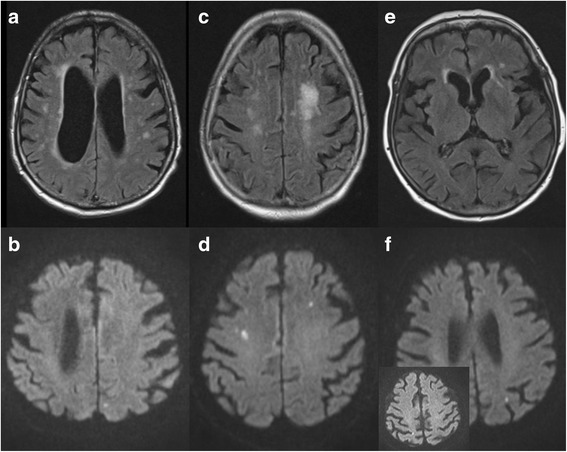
Brain magnetic resonance imaging of three patients during septic shock. The images are fluid-attenuated inversion recovery (FLAIR; a, c, and e) and echo planar imaging diffusion-weighted imaging (DWI; b, d, and f) scans. a and b An 81-year-old male patient with urosepsis. a Axial FLAIR image obtained on day 5 after the onset of septic shock shows punctiform and confluent white matter hyperintensities (WMH) in both paraventricular and paramedian regions (grade 2 leukoencephalopathy). b DWI study shows subacute ischemic lesion in the left occipital paramedian region. c and d An 80-year-old female patient with urosepsis. (c) Axial FLAIR image obtained 9 days after the onset of septic shock shows confluent WMH in the left periventricular region (grade 2 leukoencephalopathy). d DWI study shows bilateral ischemic lesions in the frontal region. e and f An 80-year-old female patient with urosepsis. e Axial FLAIR performed 8 days after onset of septic shock revealing a single punctiform WMH in the left periventricular region (grade 1 leukoencephalopathy). f DWI study shows punctiform ischemic lesions in the left occipital and parietal (*inset*) regions
